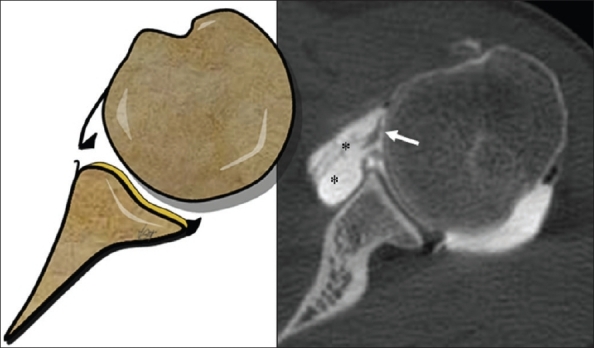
An axial CT-Arthrography image of a 32-year-old man, demonstrates the capsular lesion (*asterisks*) with the detached anterior labrum (*arrow*), which constitutes the fibrous Bankart lesion
